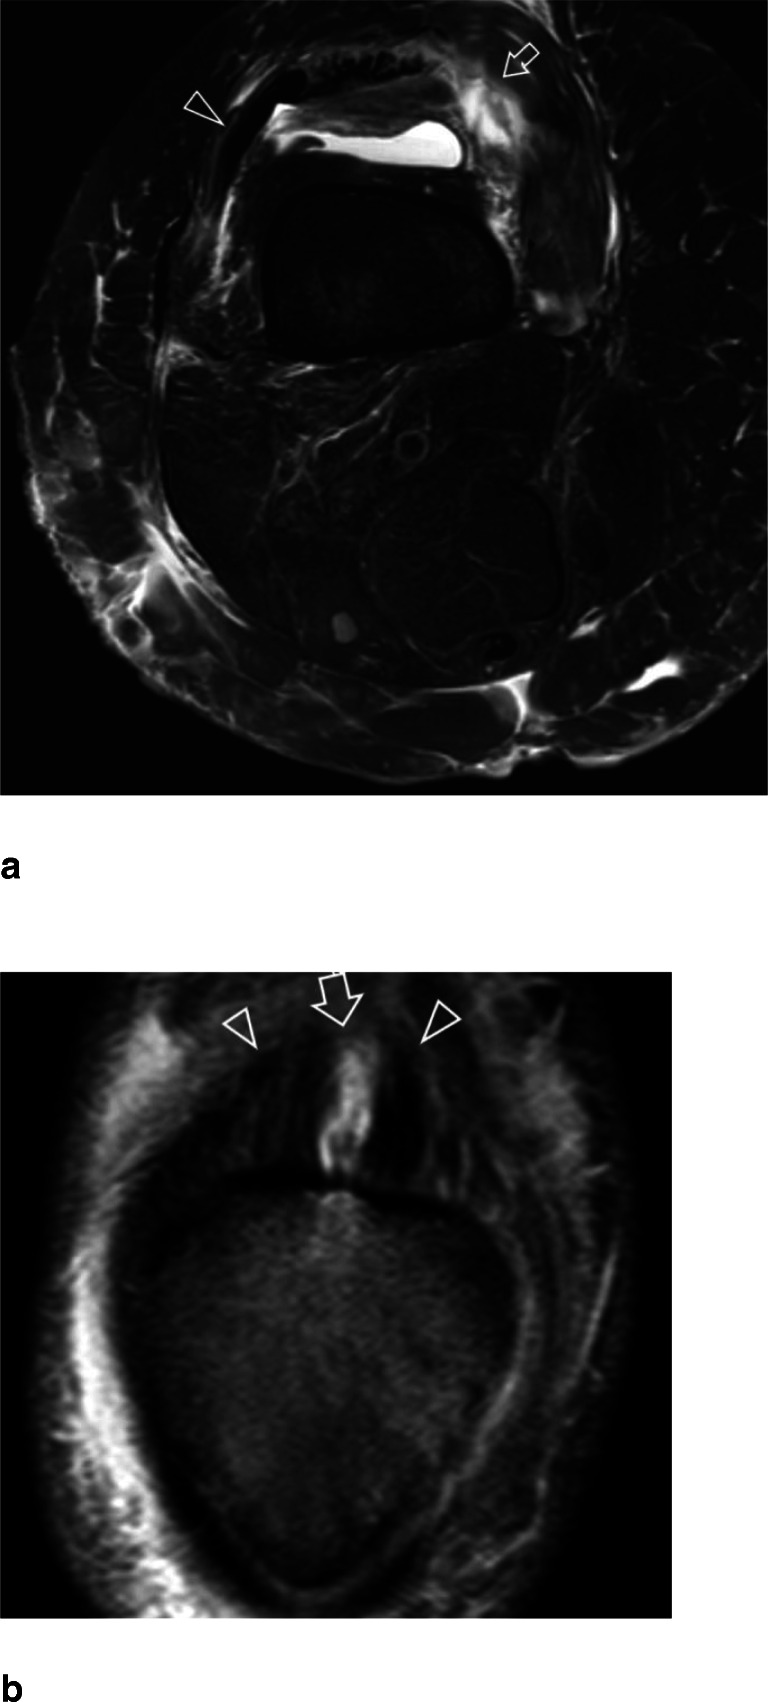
Fifty-two-year-old female with partial rupture of the right quadriceps femoris tendon (a). Axial T2-weighted MR image with fat saturation shows a complete disruption of the vastus medialis tendon (arrow), while the vastus lateralis tendon is intact (arrowhead). b Forty-seven-year-old male with partial rupture of the quadriceps femoris tendon. Coronal Intermediate-weighted MR image with fat saturation shows a partial tear of the rectus femoris tendon (arrow), while the lateral and medial portion of the tendon is intact (arrowheads)
